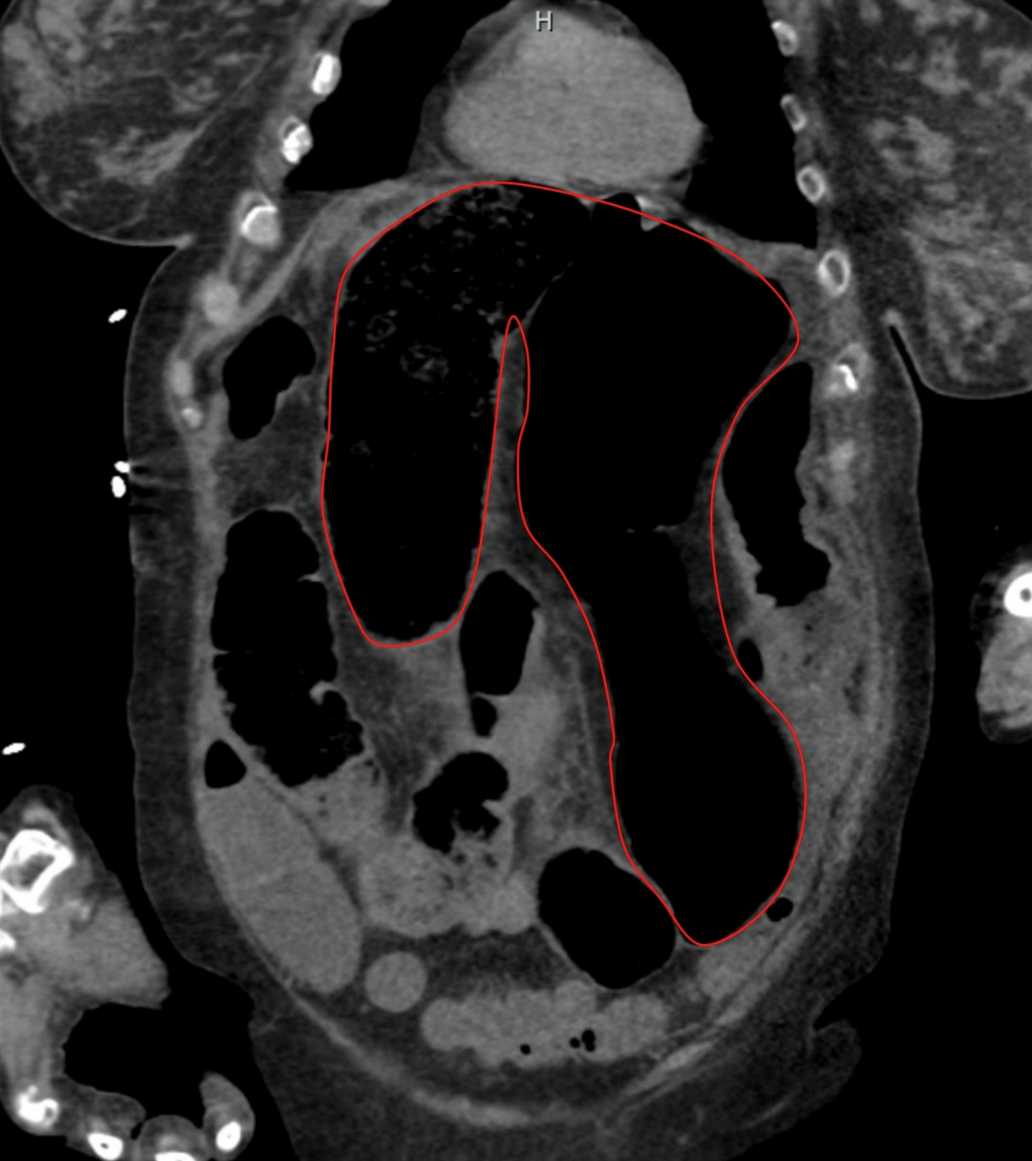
AP view of CT abdomen and pelvis without contrast showing a dilated bowel consistent with sigmoid volvulus, exhibiting the classic "coffee bean" sign, which is delineated in red. AP: anterior-posterior; CT: computed tomography
